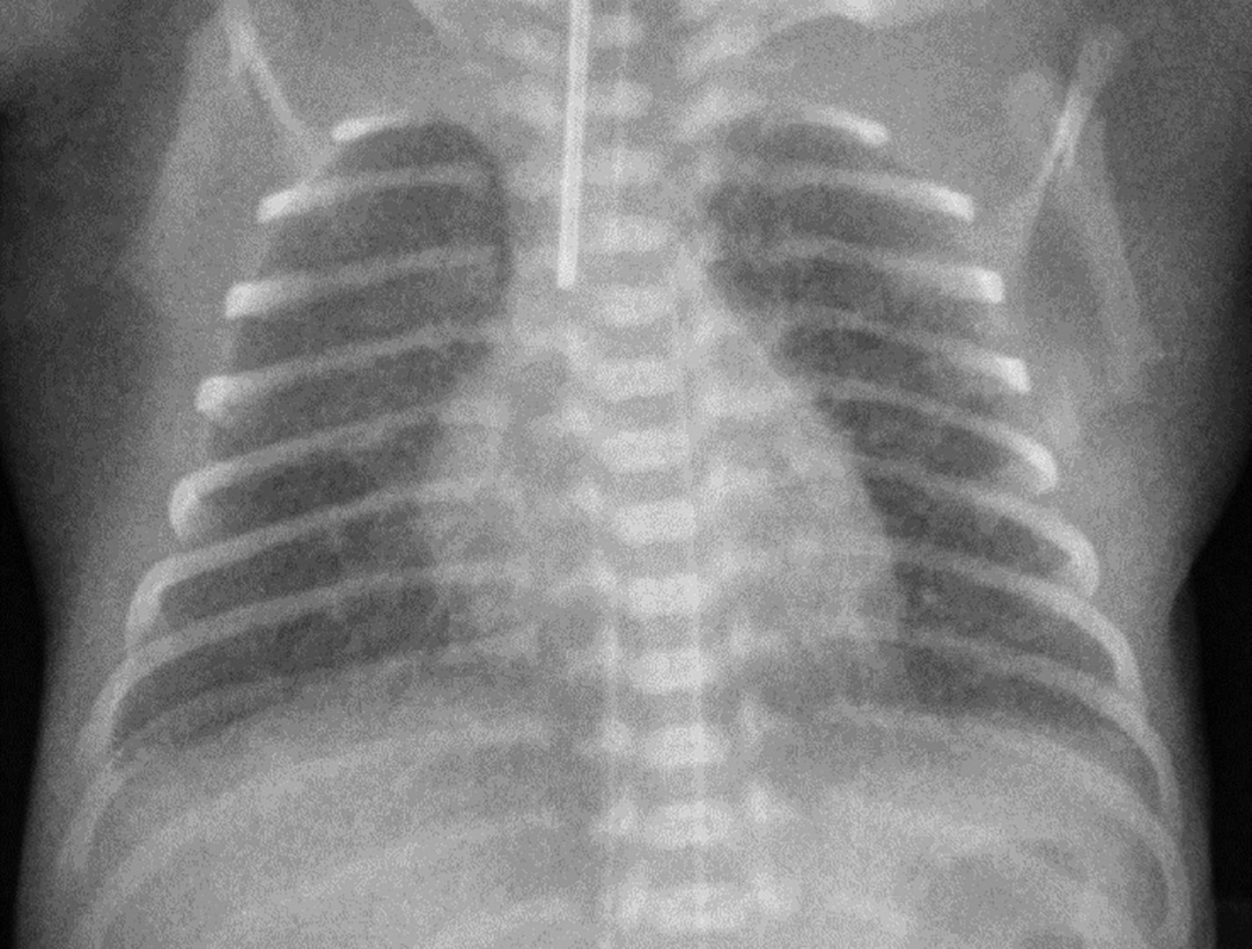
Anteroposterior radiograph shows an intubated 23-week premature infant at the 5^th^ hour of life with respiratory distress syndrome (RDS) showing pulmonary hypoventilation and granular densities bilaterally. Note the incorrect position of the tip of the arterial catheter.
